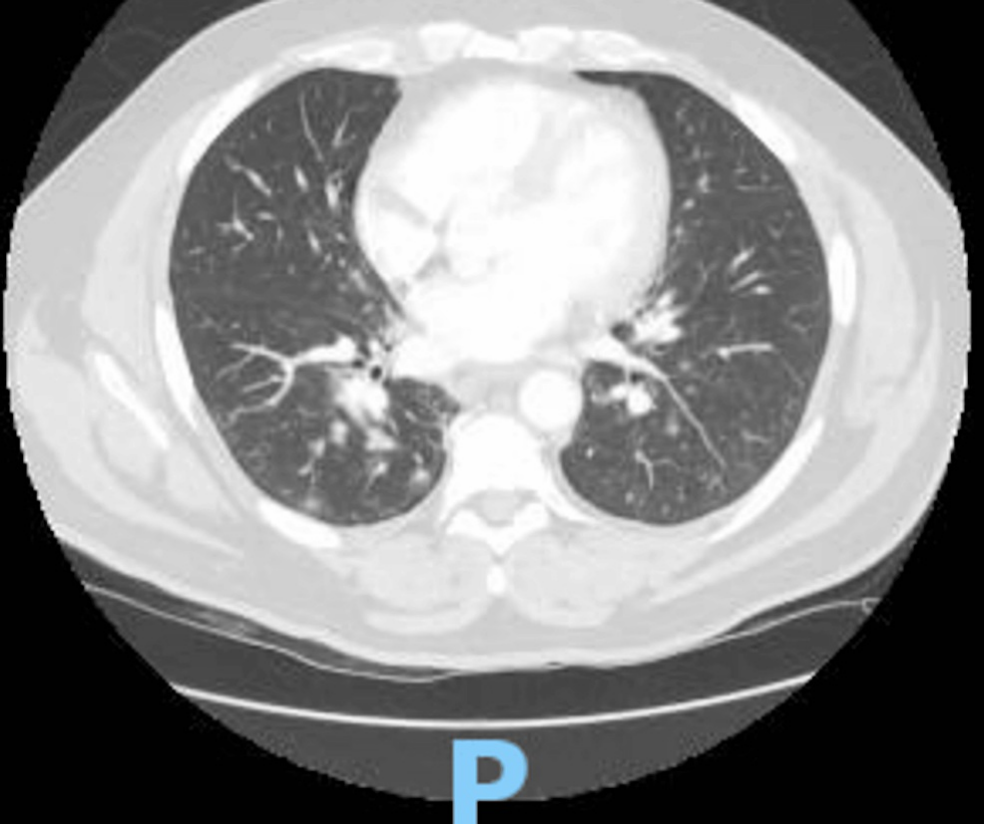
CTA scan of the chest performed on hospital day two to rule out acute pulmonary embolism demonstrating patent central airways, numerous patchy bilateral lower lobe predominant nodules/opacities.
